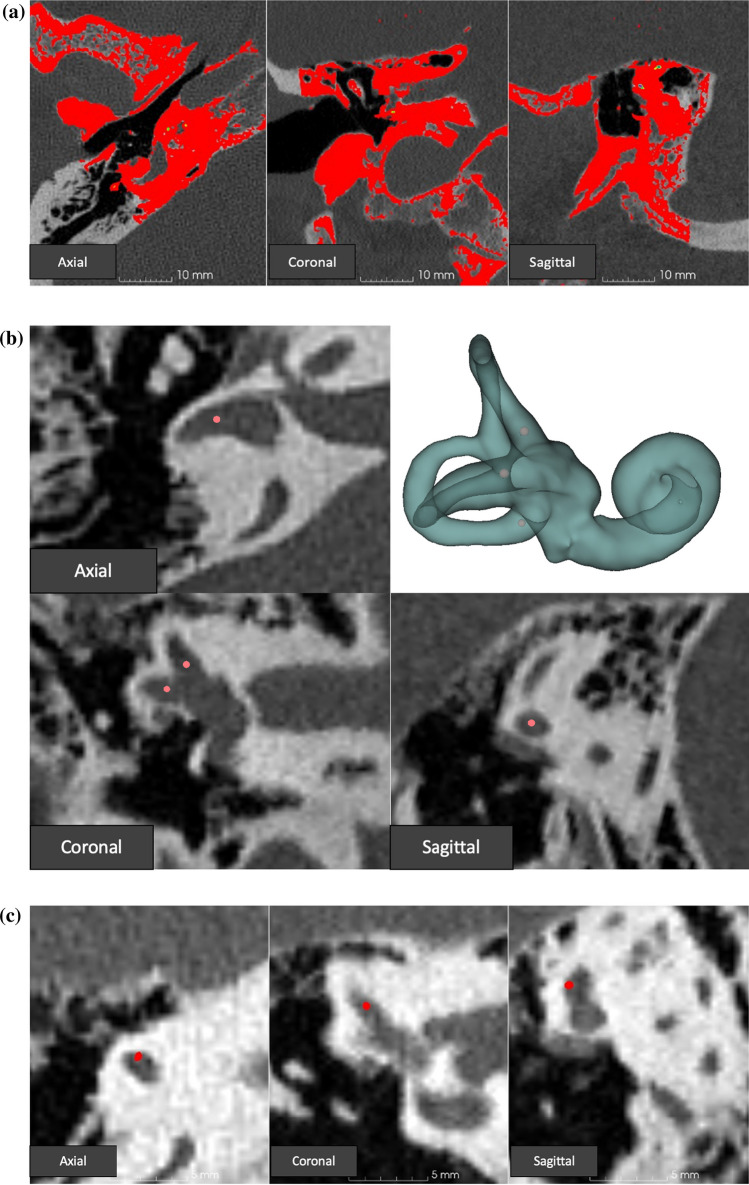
The imaging analysis process (right ear). A Manual fusion of the preoperative CT with the postoperative CT in the axial, coronal, and sagittal plane (left to right). Both images are projected in the same window with the postoperative image in front of the preoperative image. The postoperative image is colored (red) to make differences in position more clear. The position of the postoperative CT scan is manually adjusted until both scans are aligned. Next, the BRAINSFit algorithm [23] fuses the images even more precisely. B The inner ear is segmented on the preoperative CT scan in the axial, coronal, and sagittal planes. Next, a 3D rendering of the segmentation is created. Consequently, the center of the ampullae of all three canals is defined based on the 3 axes of the CT imaging as well as the 3D segmentation. A fiducial is placed at the defined centers (pink dot). 2C: The fiducials at the center of the ampullae are made invisible. Subsequently, the intensity of the postoperative CT scan (red scan of A) is adjusted until only the ball contact, which has the highest intensity (red color) is visible in all 3 planes (axial, coronal, and sagittal). A fiducial, visually enlarged to the same size, is placed in this location
